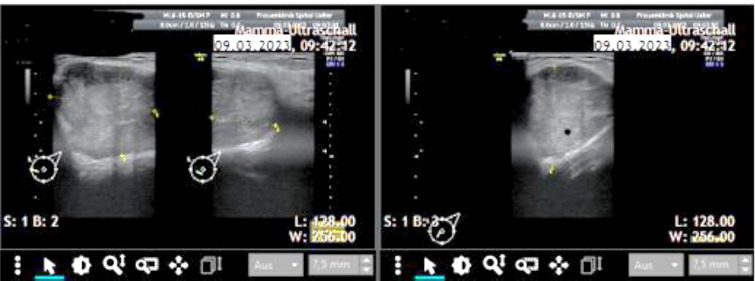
Ultrasonography results. Case 1
